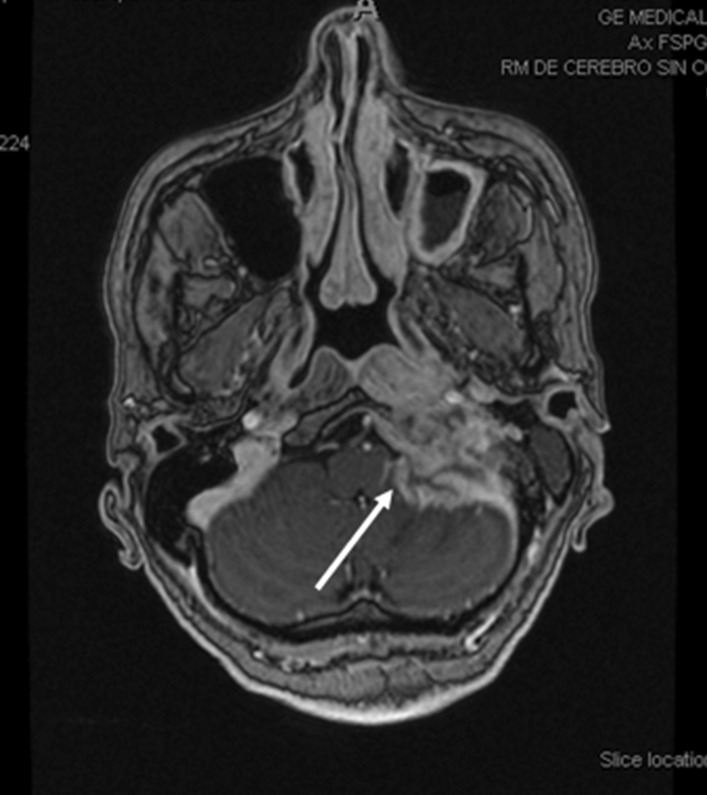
Magnetic resonance imaging June 2018 showing metastatic lesion in the skull base with destruction of bony structures
